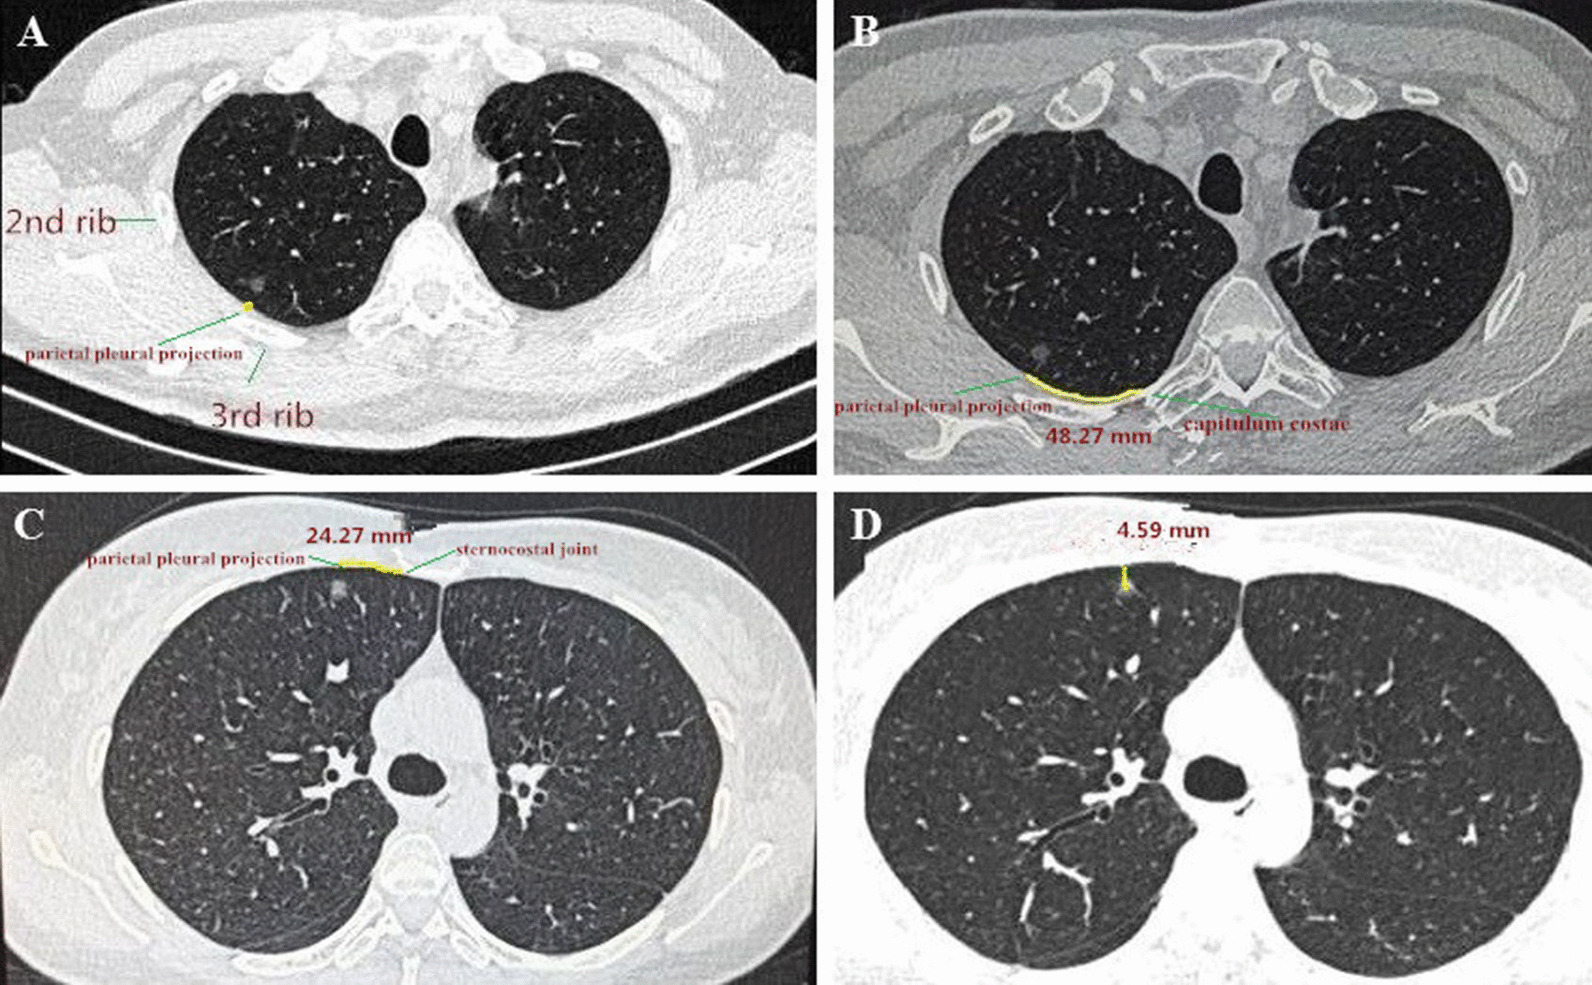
The three-dimensional geometric localization parameters of ground glass opacity (GGO) were measured according to CT imaging series before operation. A Y-axis value referred to the rib or intercostal space corresponding to the parietal pleural projection of GGO. B For GGO in the rear, X-axis value represented the measured distance between the parietal pleural projection and capitulum costae. C For GGO in the front, X-axis value represented the measured distance between the parietal pleural projection and sternocostal joint. D Z-axis value was the lesion depth from pleural surface
